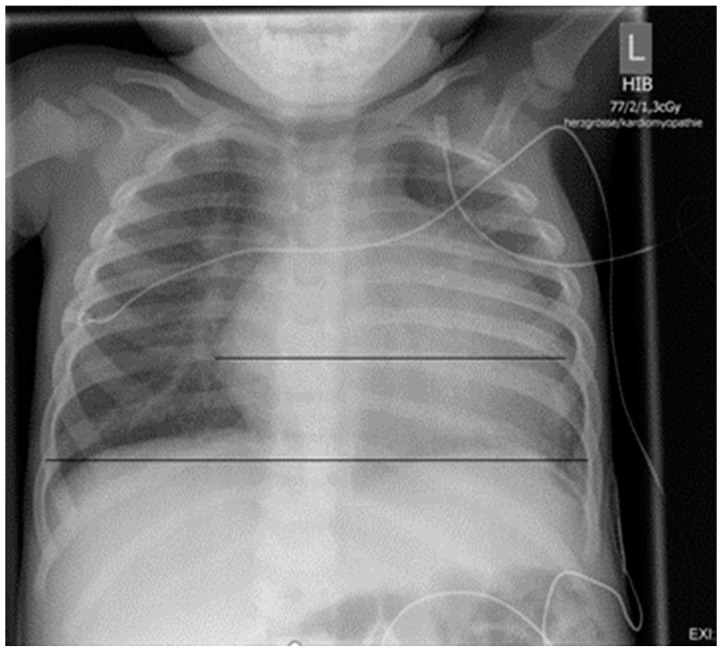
Chest X-ray on admission: cardiac silhouette is clearly enlarged (HTQ 0.64).
